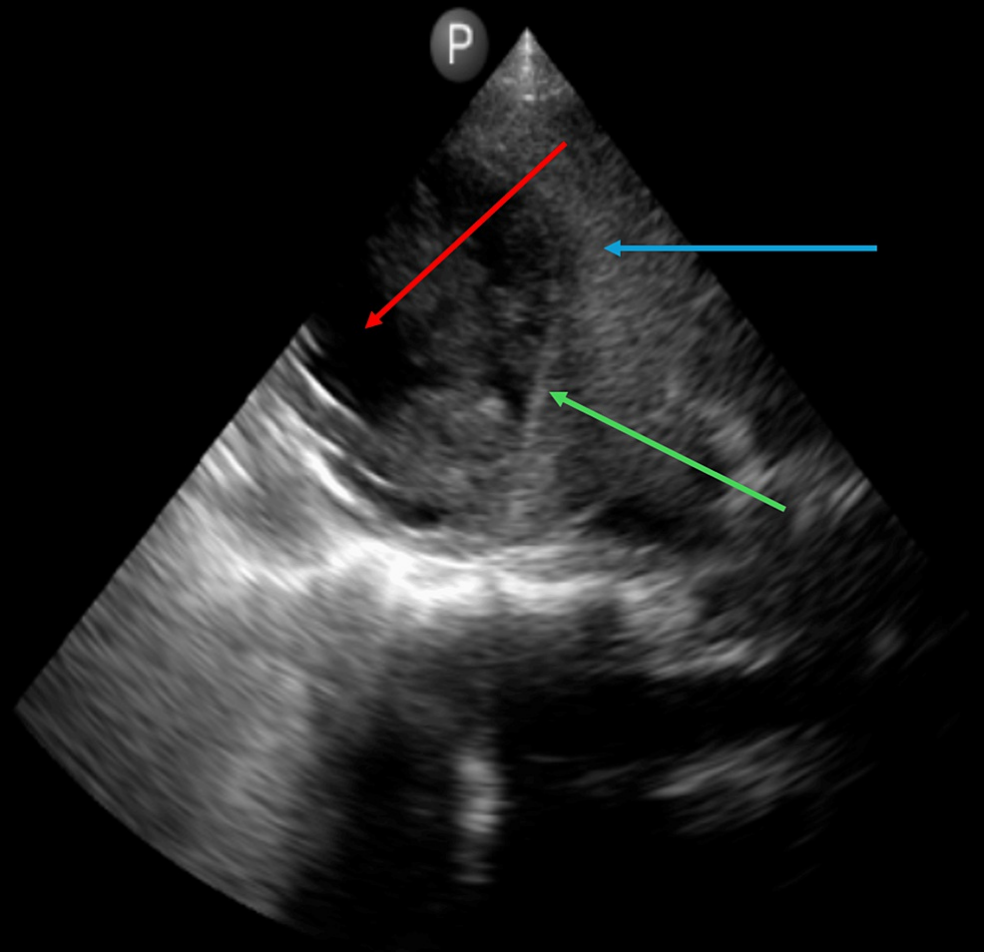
Liver POCUS image, one month after the first aspiration, demonstrating decreased abscess size. POCUS: point-of-care ultrasound. The blue arrow indicates the liver, the red arrow indicates the abscess, and the green arrow indicates the hyperechoic rim.
